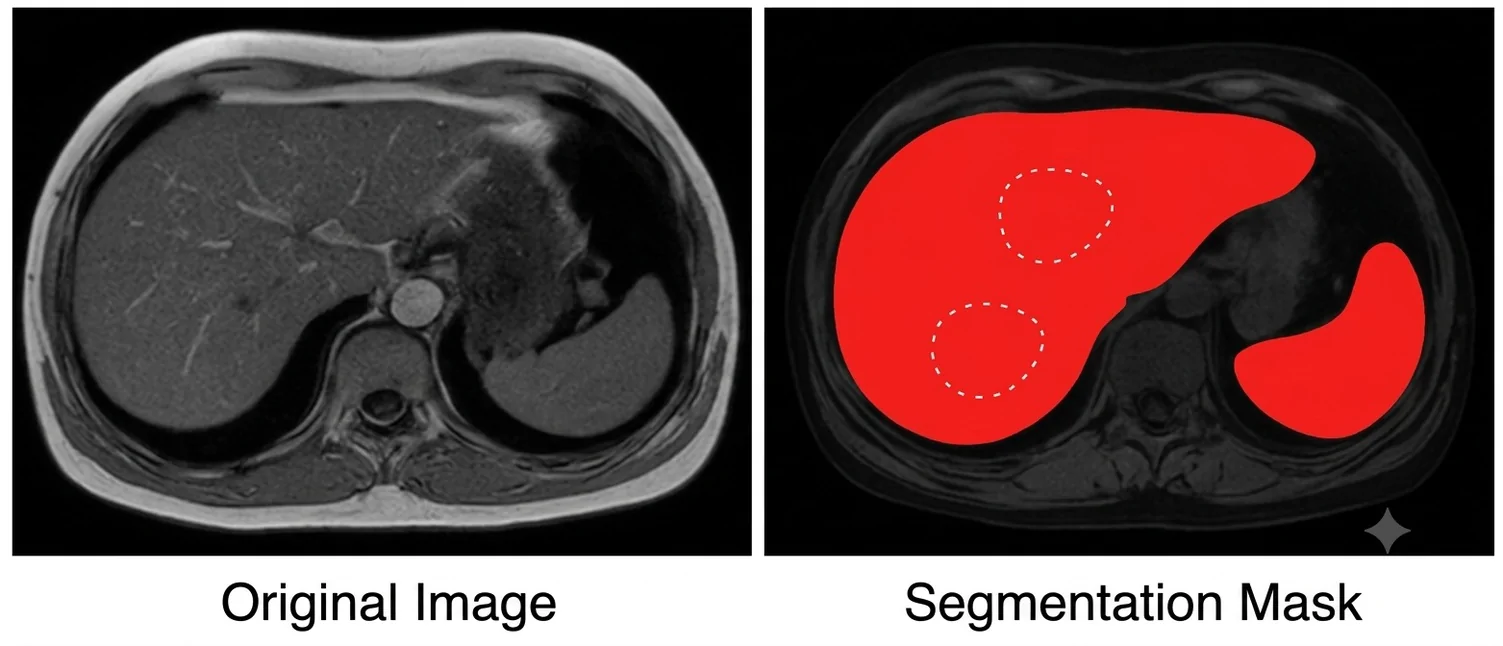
AI-aided liver segmentation using MRI.
